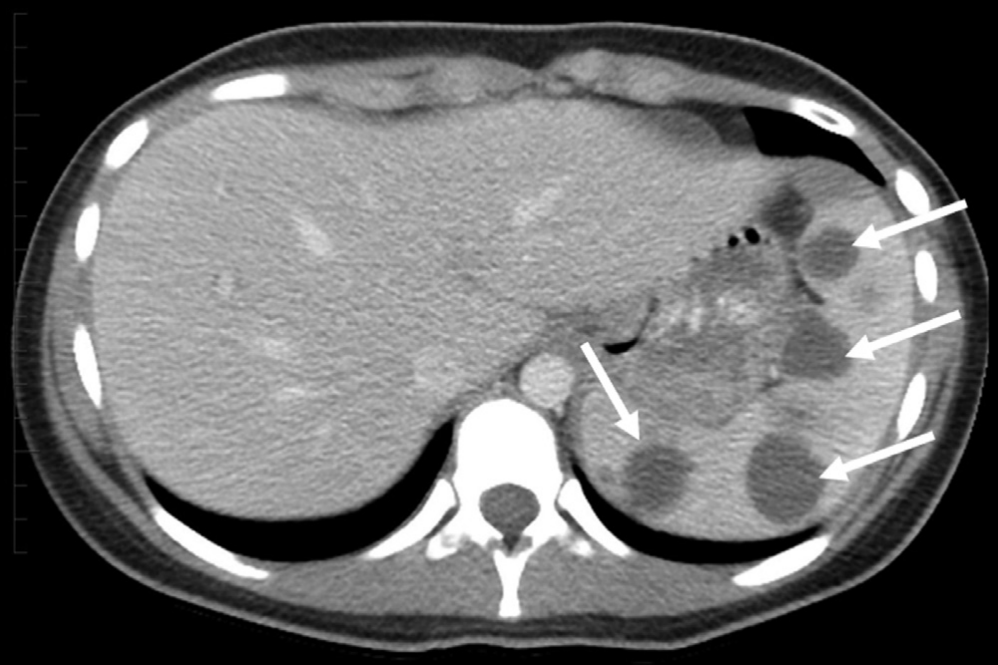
Axial CT with IV contrast demonstrating multiple round fluid collections in the spleen (arrows).
